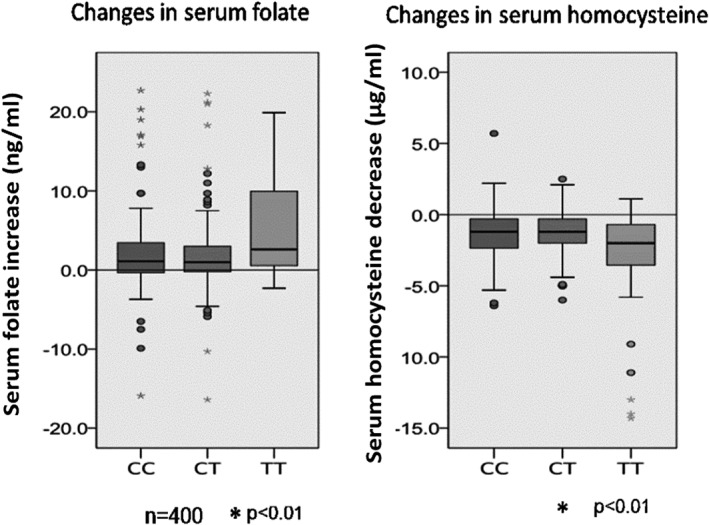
Effects of genome notification on changes in serum folate and serum homocysteine in individuals carrying three different genotypes of MTHFR (CC, CT and TT). Bars represent the means ± SD.
